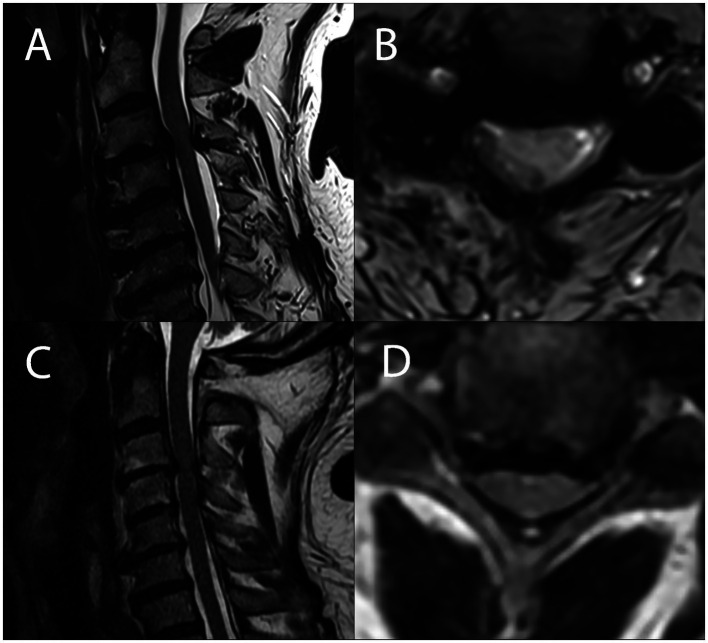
Sagittal and axial MRI sections of the cervical spinal cord showing degenerative compression in a patient with symptomatic degenerative cervical myelopathy (A,B) and in a patient without any myelopathic signs and symptoms (C,D), showing no visible difference in the severity of compression between DCM and ADCC subjects.
